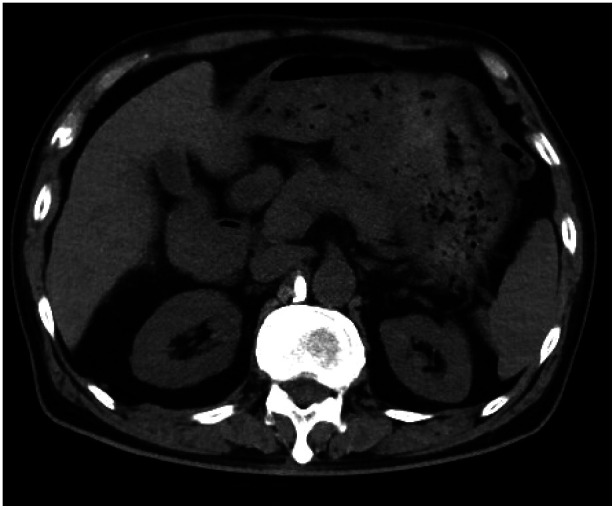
Abdominal CT showed no signs of pancreatitis and bone cement remained inside the diaphragm at 6 months of follow-up.
